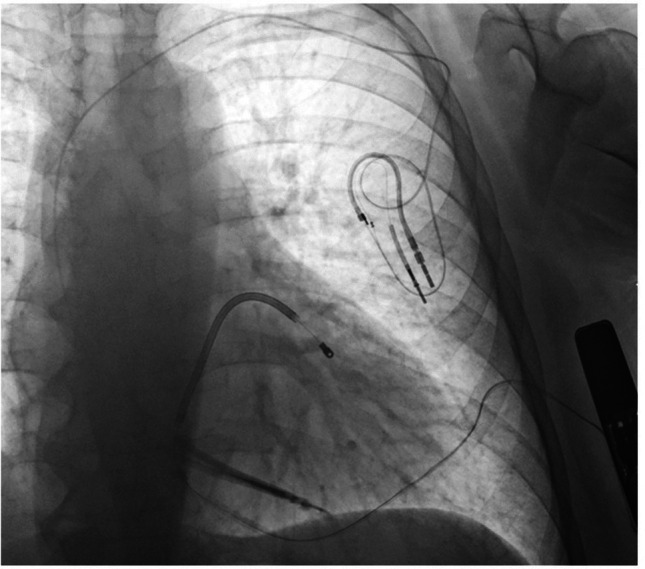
Upright X-ray image of an S-ICD recipient with extreme obesity (BMI 54 kg/m^2^). The 44-year-old female patient with idiopathic VF and a transvenous ICD implanted 14 years earlier underwent S-ICD implantation due to lead fracture. Given the high surgical risk and an occluded left subclavian vein, S-ICD implantation with abandonment of the old lead was preferred over a transvenous lead extraction. Despite successful DFT and appropriate lead positioning confirmed by intraoperative fluoroscopy, the upright chest X-ray obtained the following day revealed displacement of the distal lead tip, likely due to postural shift of excessive subcutaneous fat. The lead was subsequently repositioned using the 3-incision technique, which may have prevented the dislocation initially
